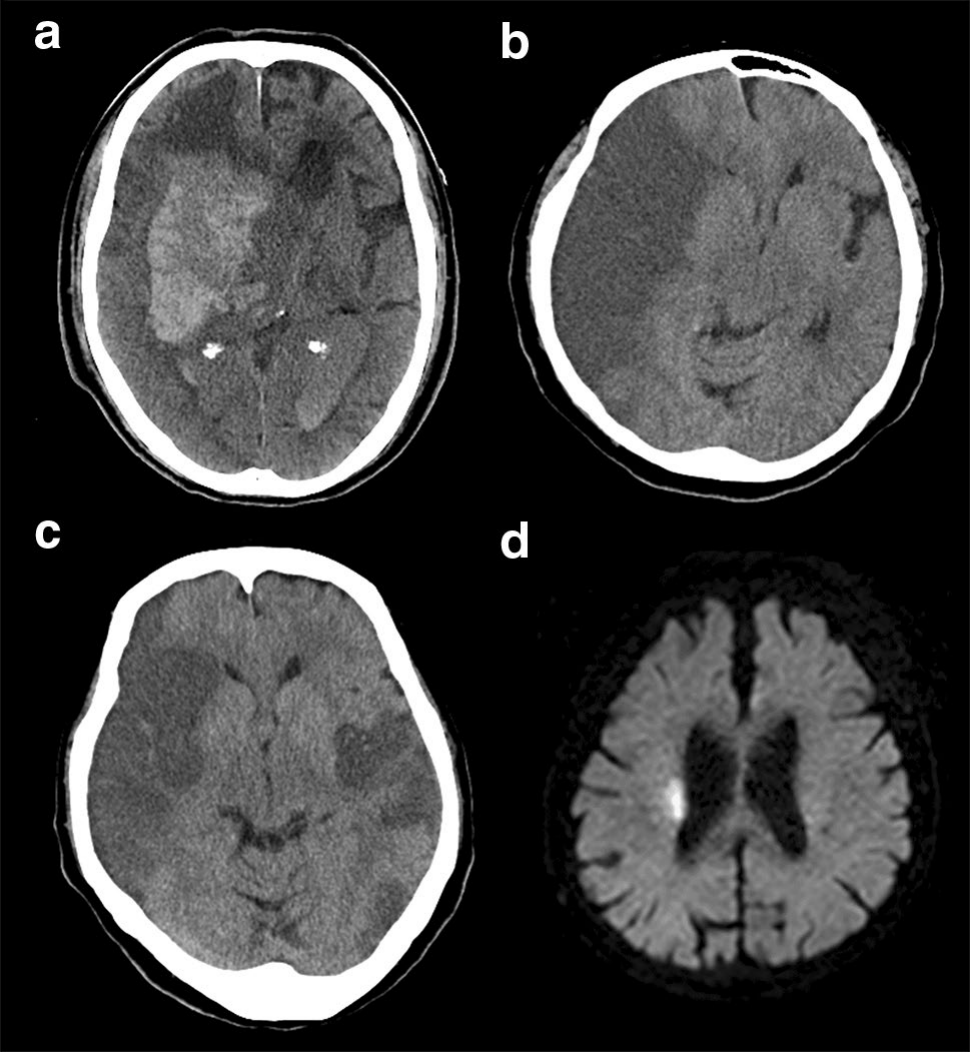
Representative brain imaging of patients with early neurological deterioration due to hemorrhagic (a) and ischemic (b–d) insults. a Axial computed tomography (CT) performed 8 h after thrombolysis showed an extended parenchymal hematoma of the right basal ganglia with additional blood in both lateral ventricles and hydrocephalus. b Axial CT performed 18 h after thrombolysis showed extensive brain edema and midline shift. c Axial CT performed 12 h after thrombolysis in a patient who became comatose during thrombolysis for ischemic stroke in the left middle cerebral artery (MCA) territory showed new acute ischemic lesions in the right MCA territory. c Diffusion-weighted imaging obtained 12 h after thrombolysis showed a hyperintense lesion in the territory of lenticulostriate arteries, which did not change much from baseline
